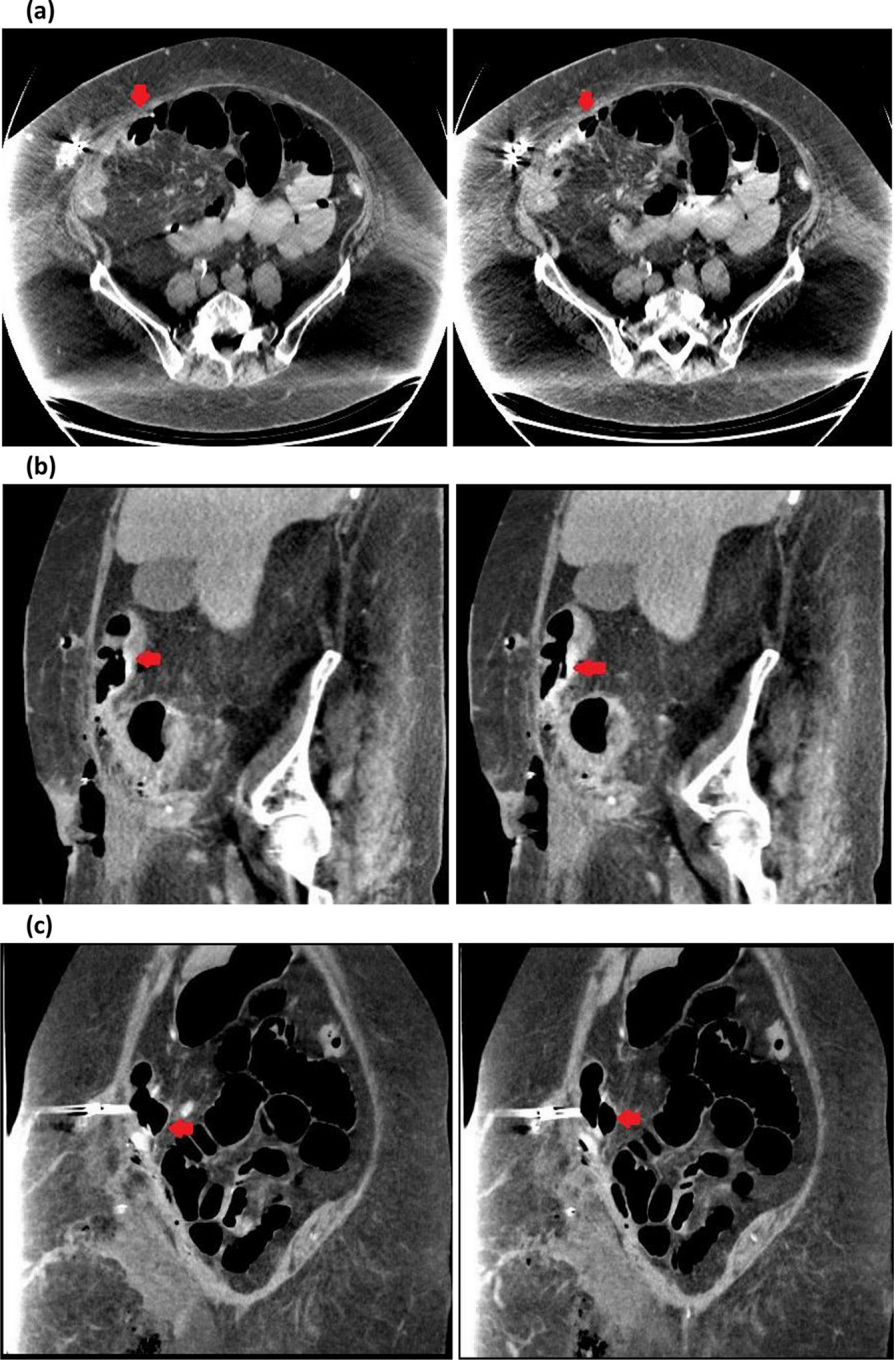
Axial (a), coronal (b) and sagittal (c) CT images showing laparoscopic-adjustable gastric band tubing (red arrow) penetrating through the transverse colon. The previous port site containing surgical clips can also be seen on axial images.
